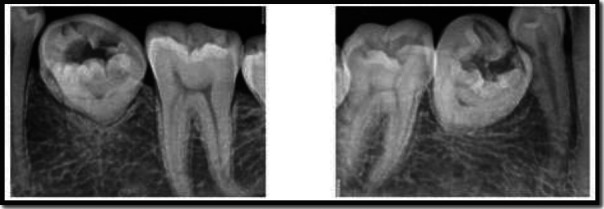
Intraoral periapical radiographs of the patient depicting unusual morphology of mandibular right and left second premolar
